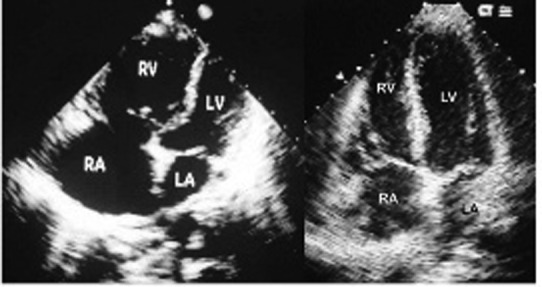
Echocardiogram in apical four chamber view. Left: Severe RV and RA dilatation. Right: Mild RV and RA dilatation in recovery.
